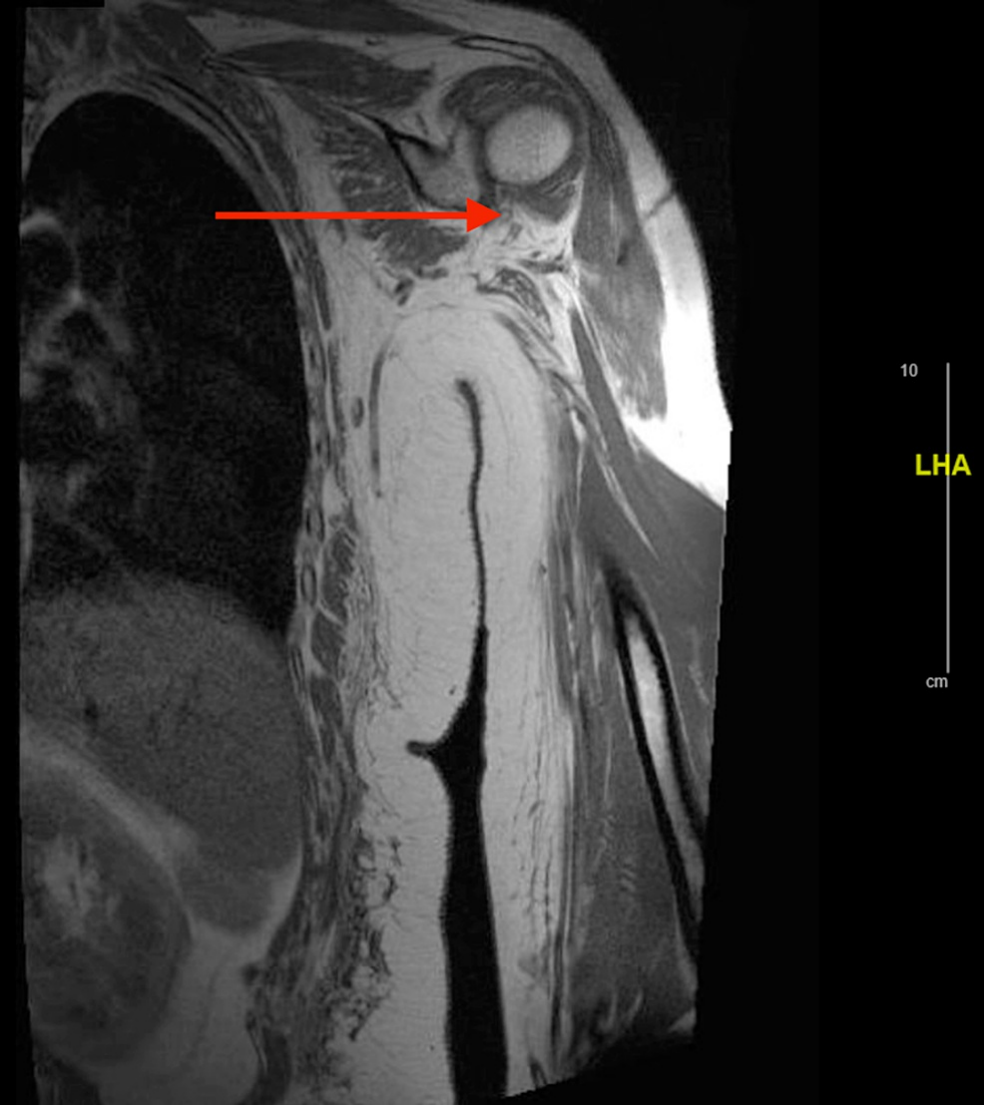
Coronal MRI of left shoulder Arrow demonstrates muscular edema of the shoulder girdle.
